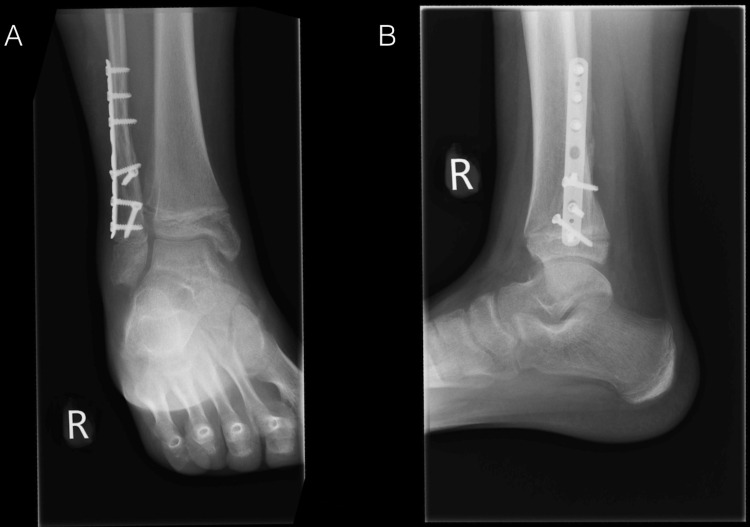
Post-operative anteroposterior and lateral X-rays of the right ankle. Six weeks follow-up. Fibular plate and screws in situ. Good position of the fractures with evidence of healing.
